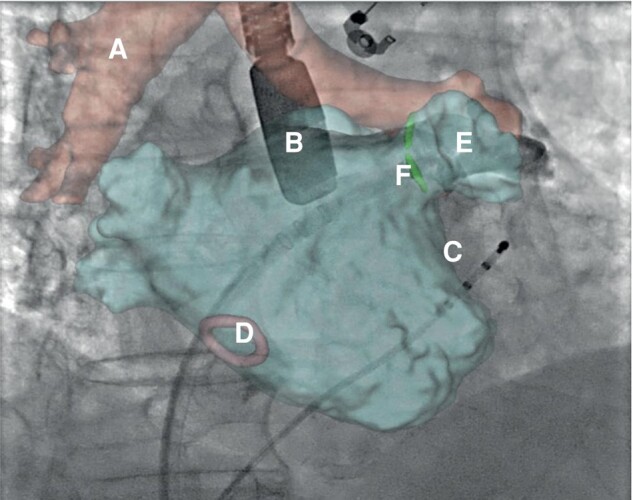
Fluoroscopy image with a 3D reconstructed CT-scan image fusion in order to guide LAA occluder positioning and deployment. A: tracheal landmark used for the fusion between the CT-scan image and the fluoroscopy system; B: transoesophageal echocardiography probe used to guide the LAA occluder positioning; C: quadripolar catheter placed inside the coronary sinus in order to guide the transseptal puncture (optional); D: transseptal puncture area; E: left atrial appendage (LAA) in right anterior projection; F: catheter positioned in front of the LAA entrance before occluder release.
